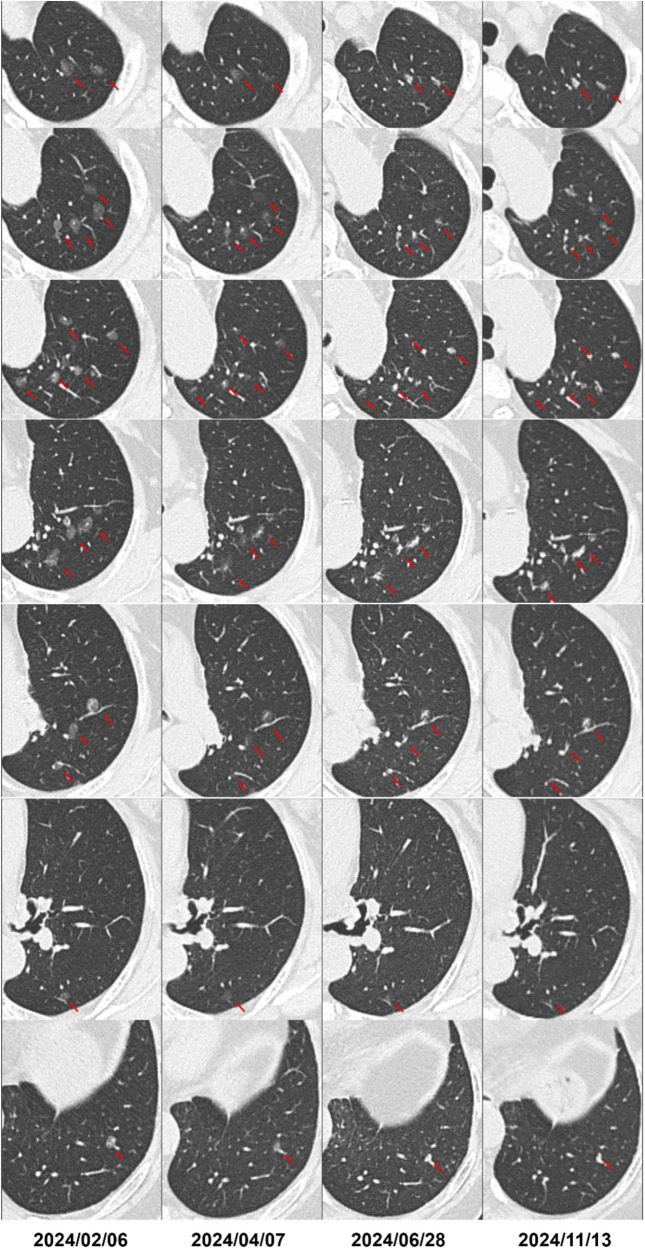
Following administration of EGFR-TKI, the multiple GGNs in the left lower lobe demonstrated a gradual decrease in size and density over time, eventually manifesting as a solid scar on chest CT.
